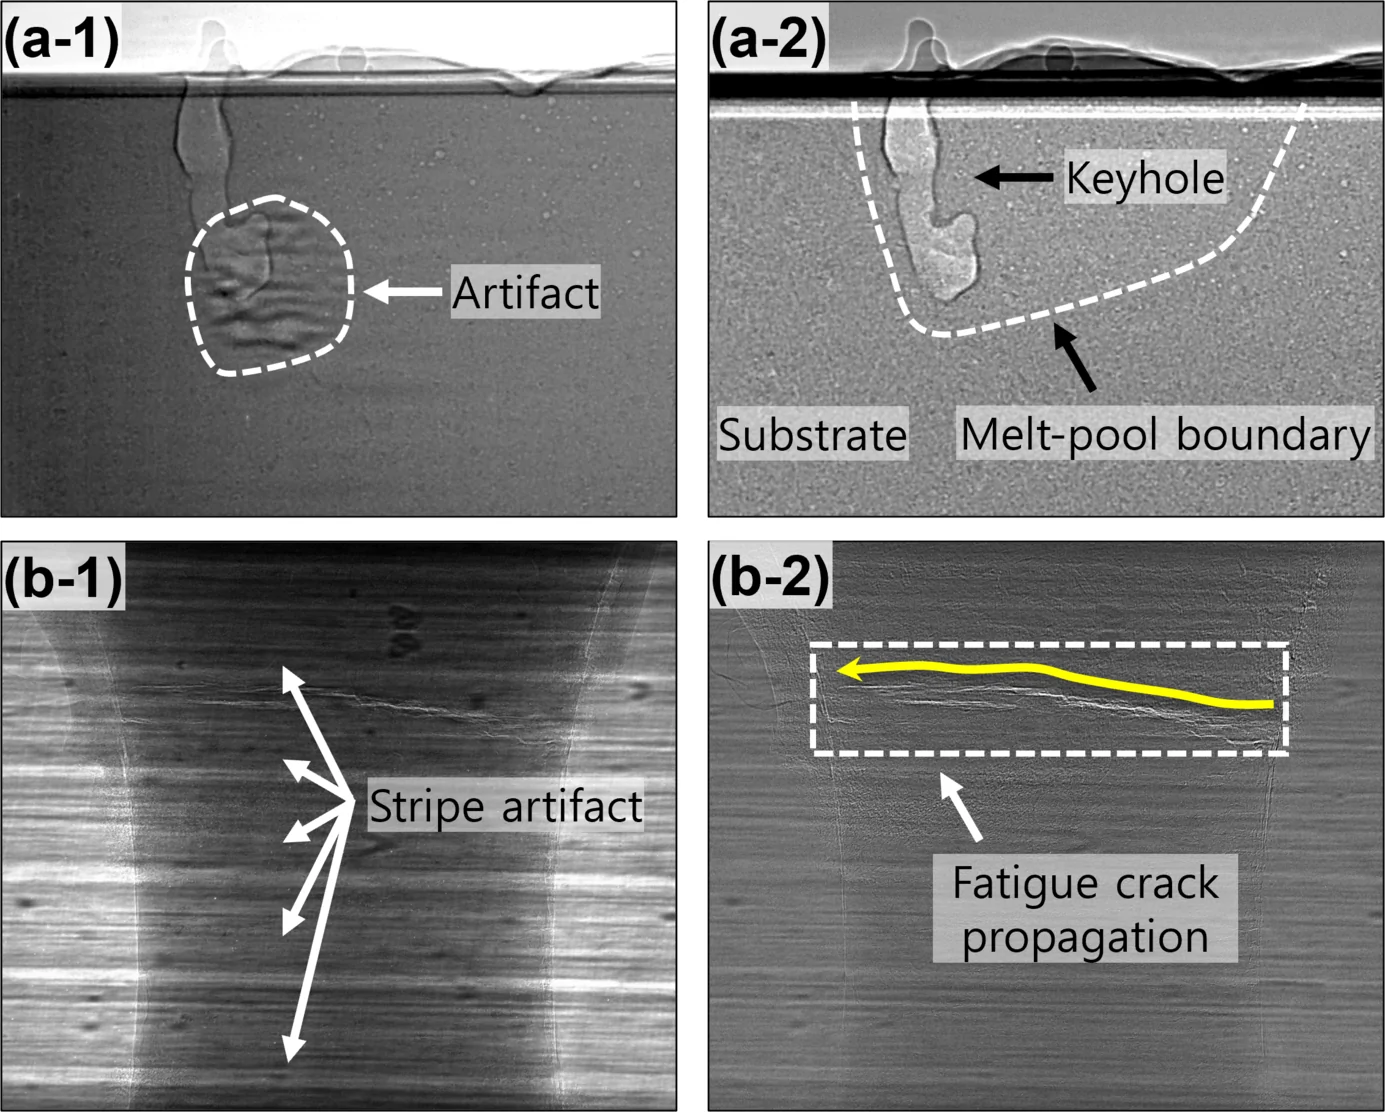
Efficacy of *SMAXI*’s gray-scale intensity normalization module. (*a*-1) A raw high-speed X-ray image of an L-PBF process of Ti-6Al-4V plate sample, exhibiting low contrast and background artifacts. (*a*-2) The normalized frame demonstrating sharpened image boundaries that clearly isolate the keyhole-shaped vapor depression and the surrounding melt pool. (*b*-1) A raw projection image of *in situ* CT experiment on fatigue corrosion of Al7075, with horizontal stripe artifacts present (Stannard *et al.*, 2017 ▸). (*b*-2) The pre-processed output, successfully flattening the illumination gradient to reveal the underlying fatigue crack propagation.
